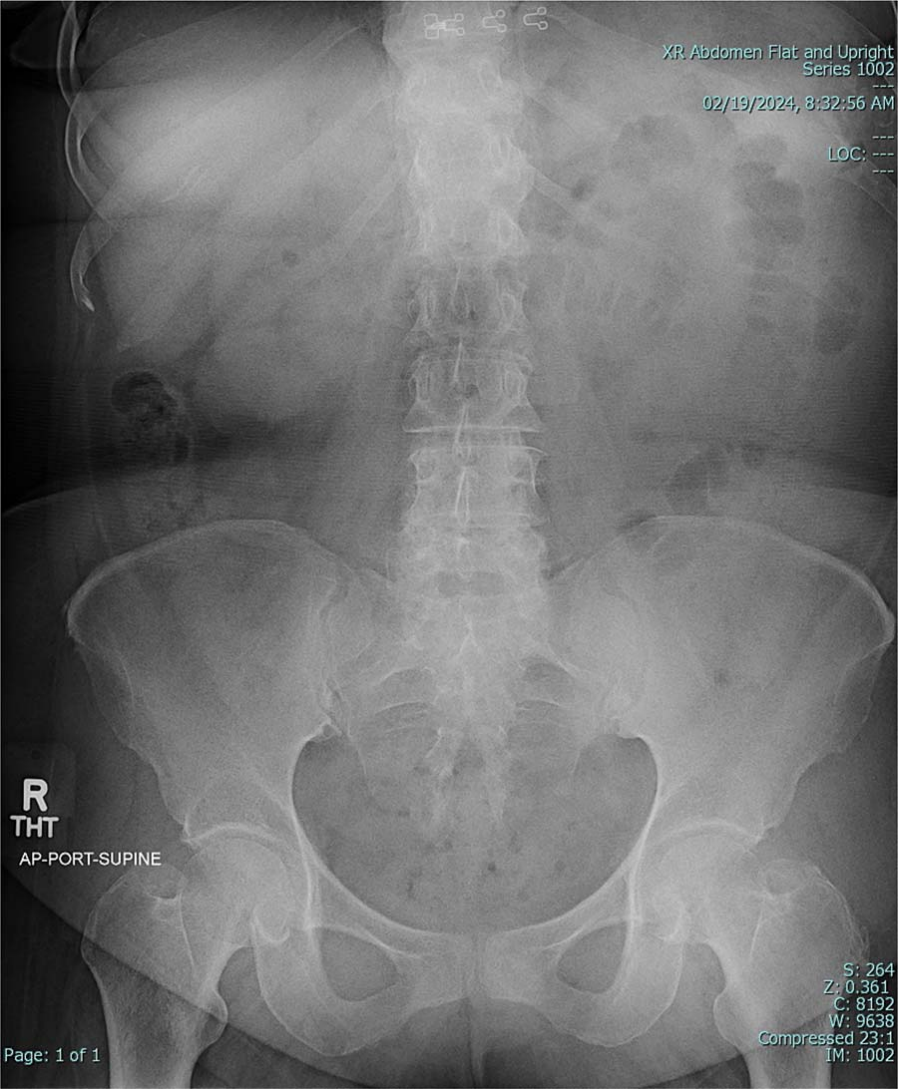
Preoperative X-ray of abdomen, demonstrating small bowel obstruction.
